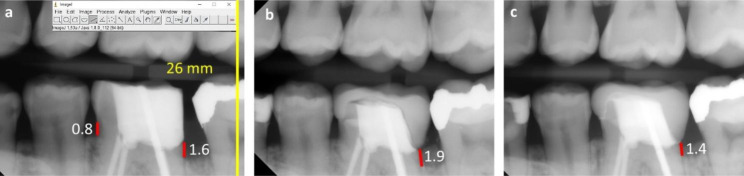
Example of crestal bone level measurements (mm) using ImageJ program. (a) A radiograph was opened in the ImageJ program. The known length (26 mm) of the sensor was used to calibrate the image. Crestal bone level measurements on the deep caries and non-caries sides before the CLP. (b) Crestal bone level measurement on the caries side at crown delivery. (c) Crestal bone level measurement on the caries side at the 12-month follow-up
